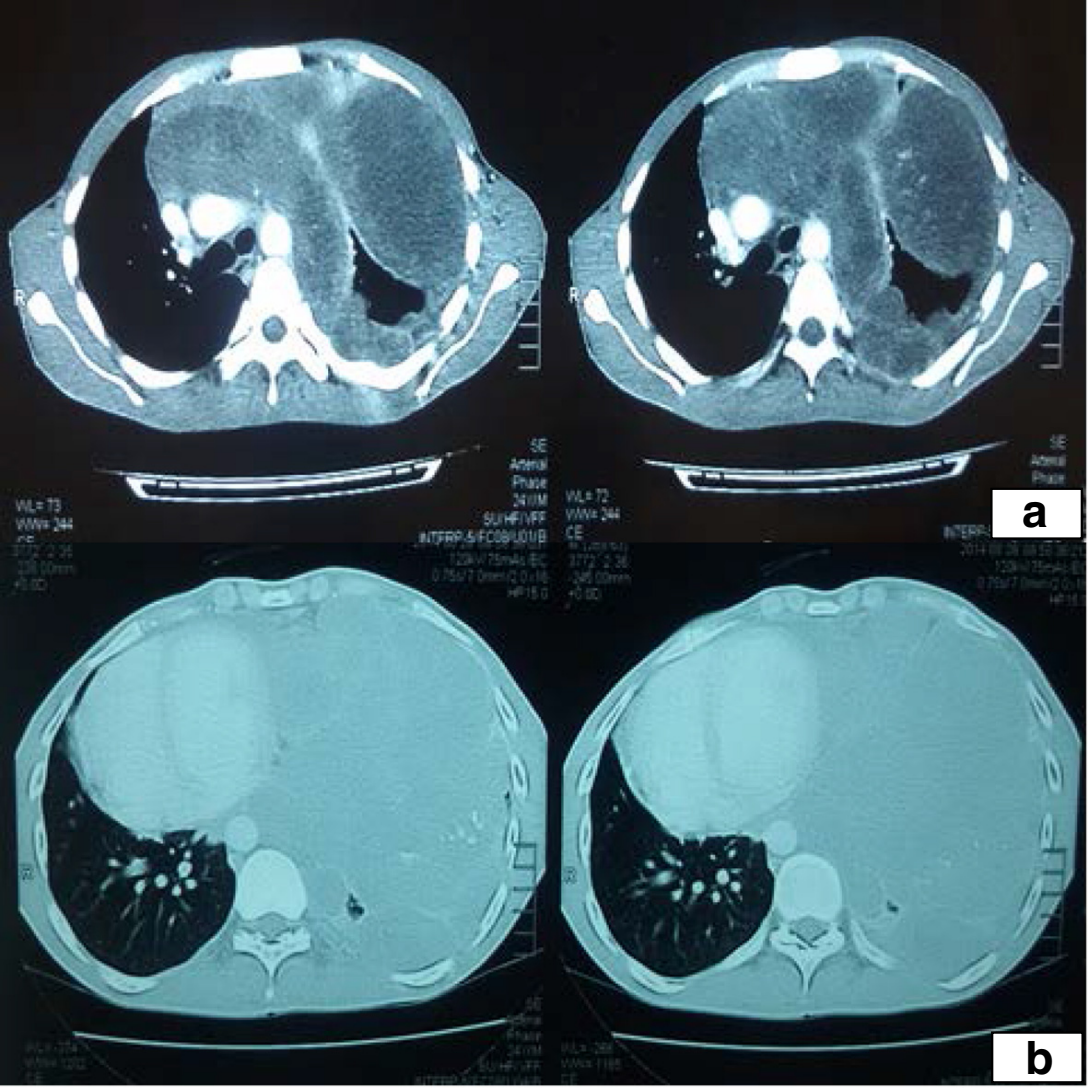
CT scan a & b: showing large heterogeneously enhancing mass effacing the left hemithorax and anterior mediastinum
